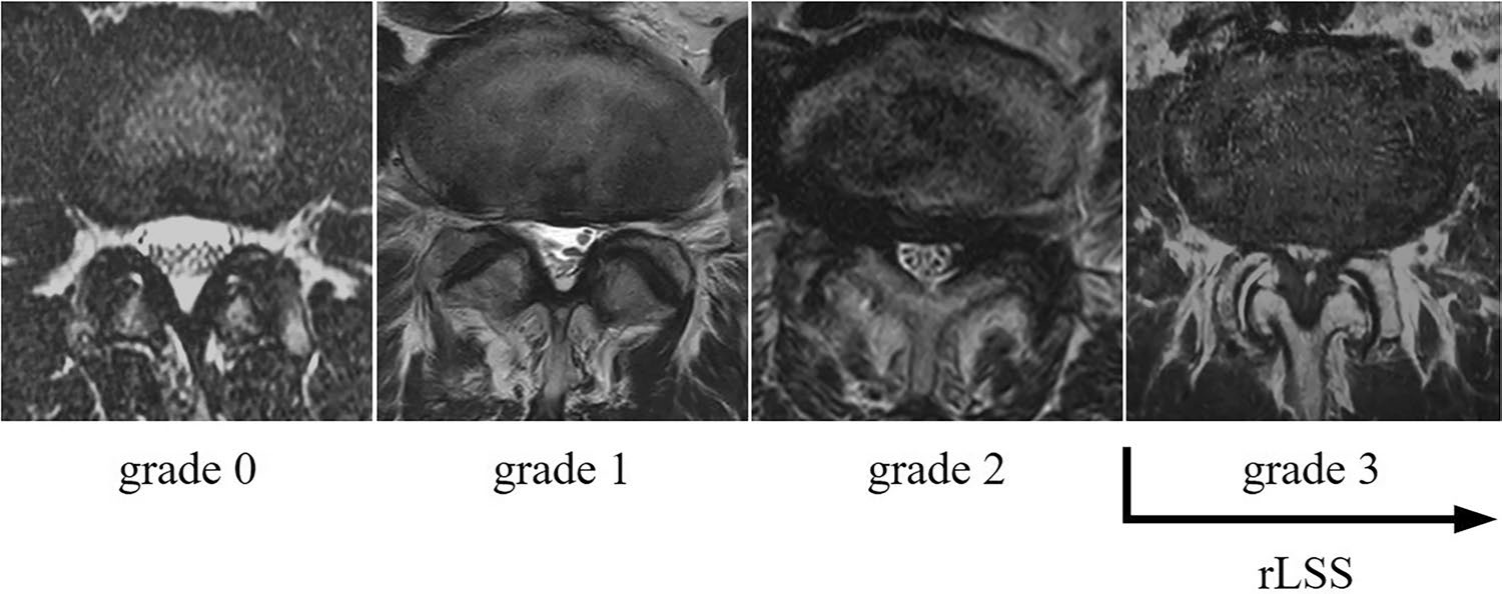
Measurement figures of rLSS (radiographic lumbar spinal stenosis). rLSS was defined as grade 3 at the most severely affected lumbar intervertebral disc level.
